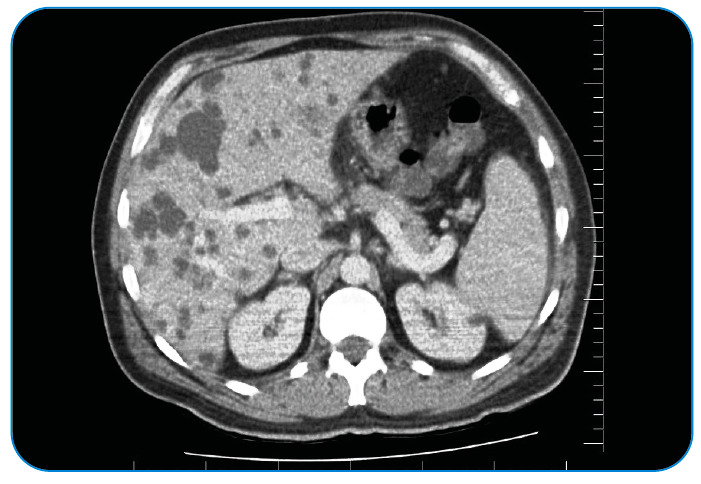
Multiple hepatic abscess in the abdominal CT scan.
